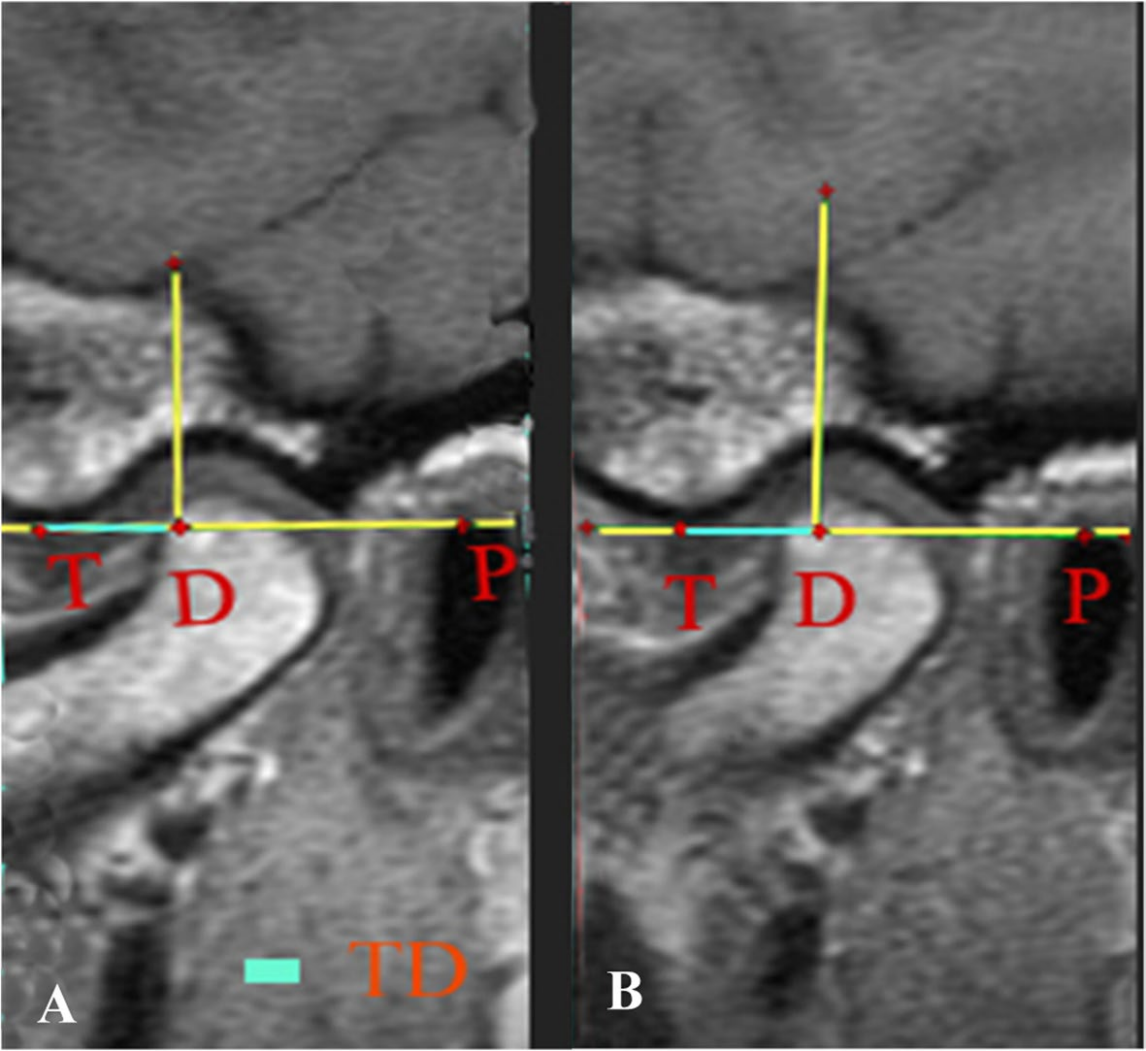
MRI showing the improvement in disc position. A. Before LLLT, B. After LLLT
